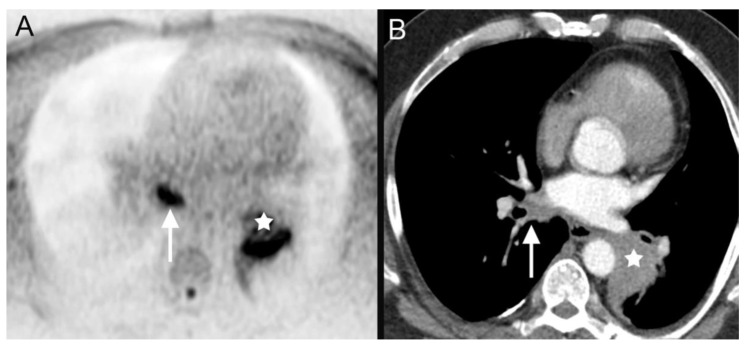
DWIBS (A) as well as contrast-enhanced thoracal CT (B) depicts the primary cancer lesion (A,B: white star) as well as the nodal metastases (A,B: white arrows) in a 60-year-old male patient who was diagnosed with a non-small cell lung cancer (cT2b cN3 cM0) in the diagnostic work-up of clinically suspected head and neck carcinoma.
